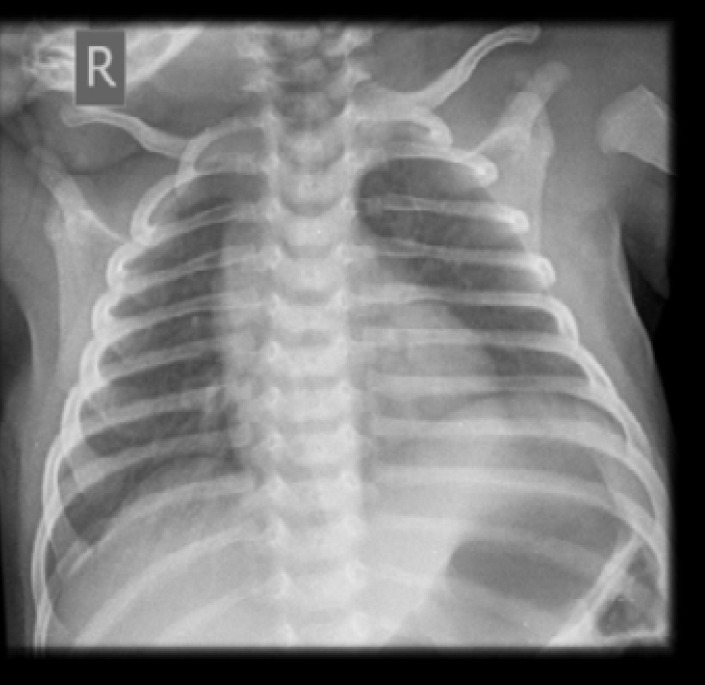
Chest X-ray on 10th day of life showed total resolution of pneumothorax and re-expansion of the right lung.
